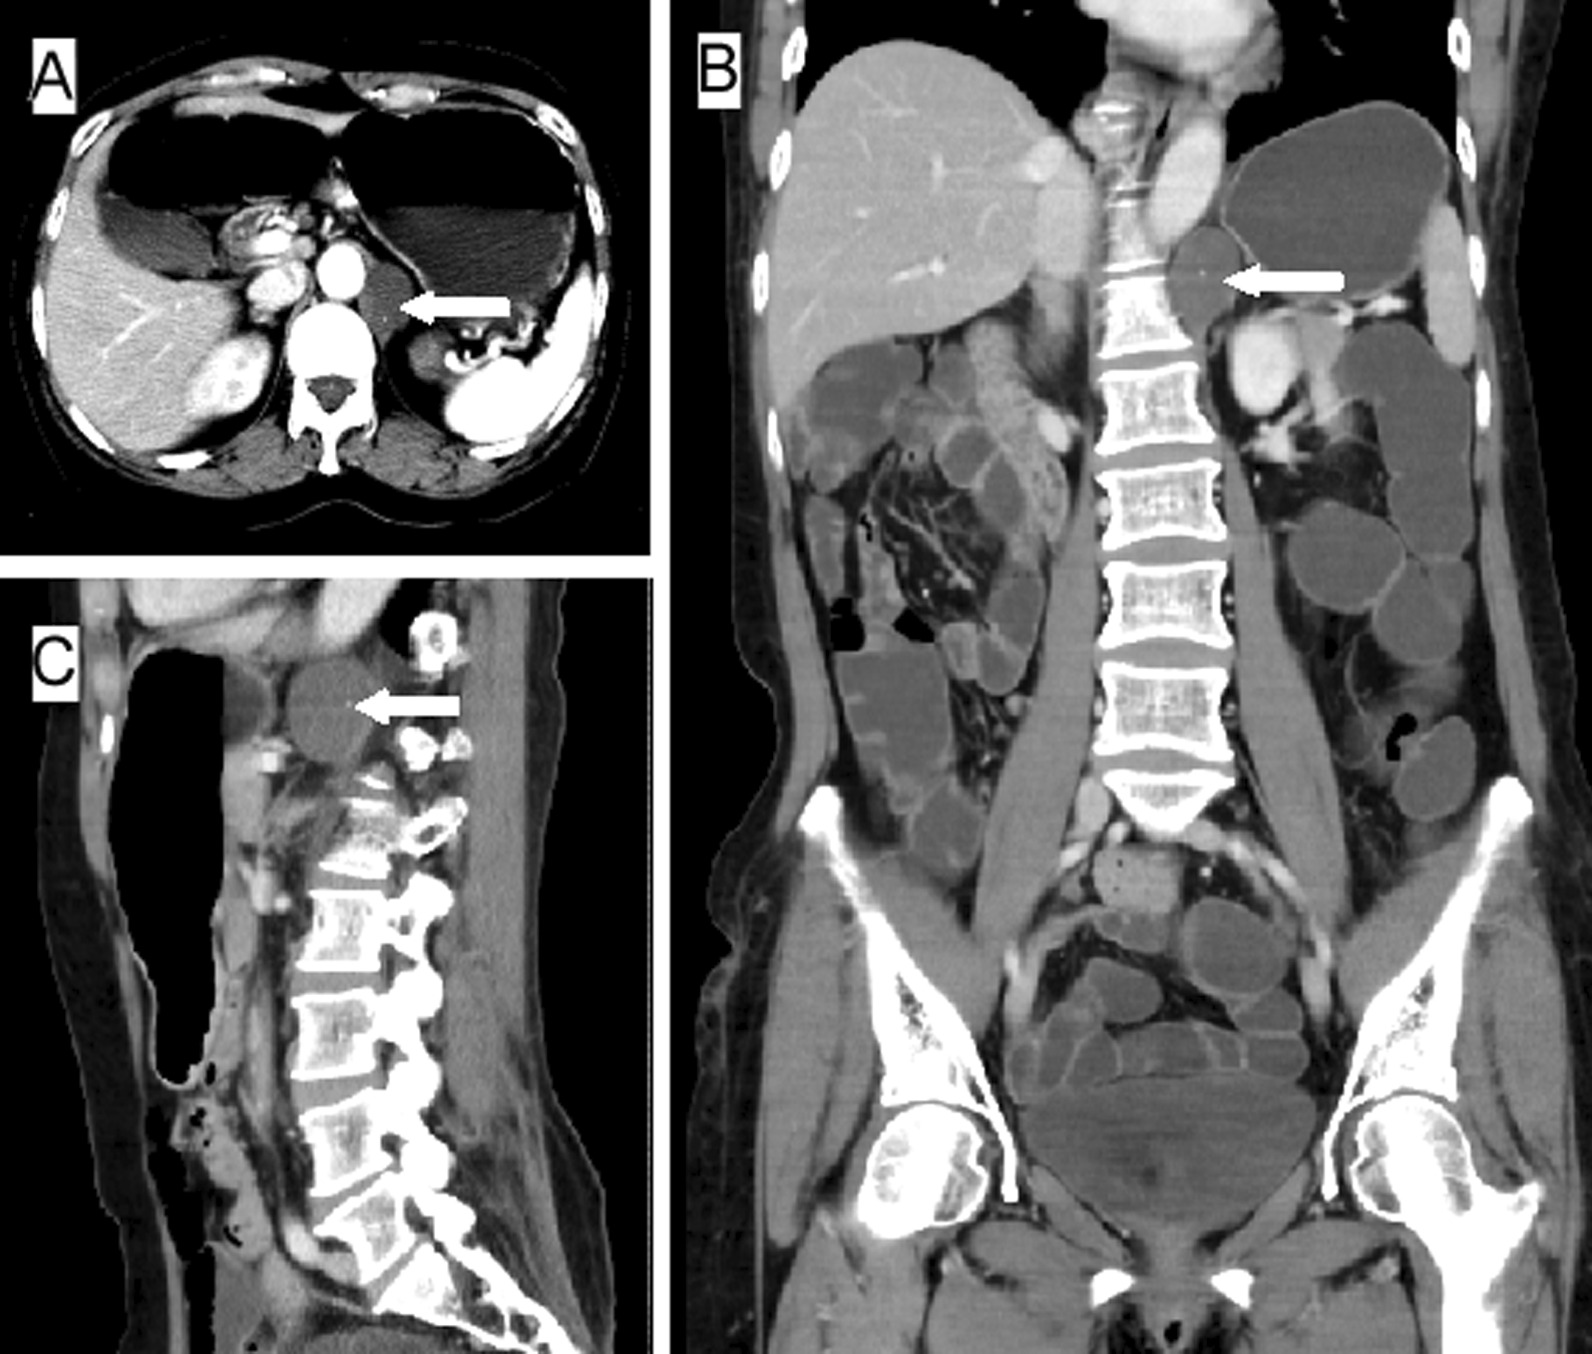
Radiological findings of the reported case. Contrast-enhanced CT scan shows a thin-walled water-attenuated cystic lesion in retroperitoneal region (white arrows). Panel A, Axial view; Panel B, Coronal view; Panel C, Sagittal view
